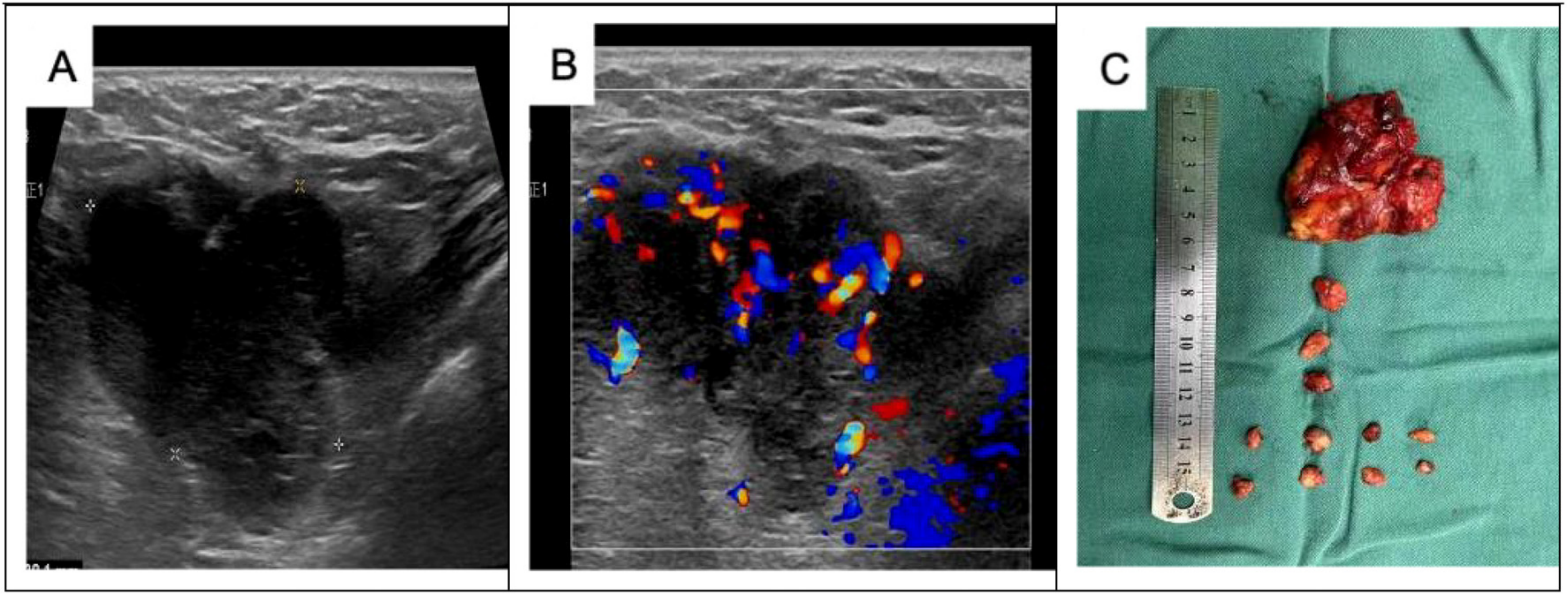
Preoperative ultrasound and postoperative images of the right axillary mass: (A) a 30.2 mm × 33.0 mm × 54.3 mm weakly echogenic mass detected in the right axilla, with poorly defined borders, irregular morphology, and uneven internal echogenicity. (B) Rich blood flow signals are detected inside the CDEF, with tortuous and irregular internal vascularization. (C) Thirteen axillary lymph nodes were cleared during the operation. Postoperative pathology revealed one cancer metastasis, while the remaining 12 lymph nodes showed reactive hyperplasia.
